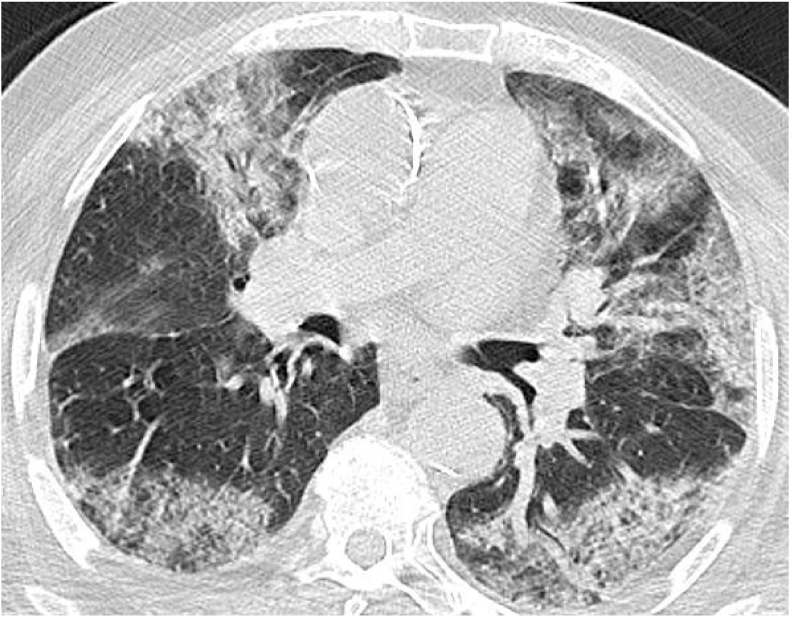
Axial no enhanced high-resolution CT (lung window) demonstrates extensive ground-glass opacities in a peripheral and central distribution, typical appearance of COVID-19 pneumonia, with more than 75% of the lung involved.
